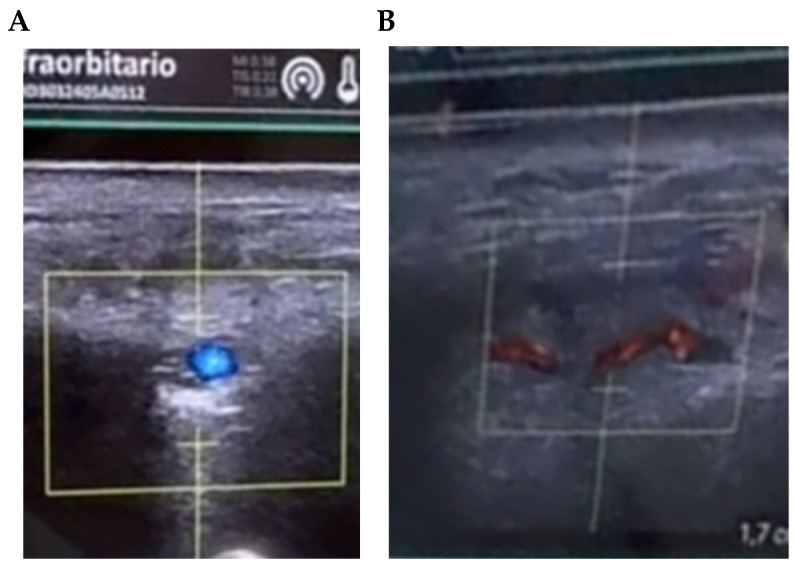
(A) Ultrasound image showing extrinsic compression of the infraorbital artery caused by hyaluronic acid deposits. (B) Ultrasound image demonstrating occlusion of the anterior superior alveolar artery consistent with retrograde embolization.
